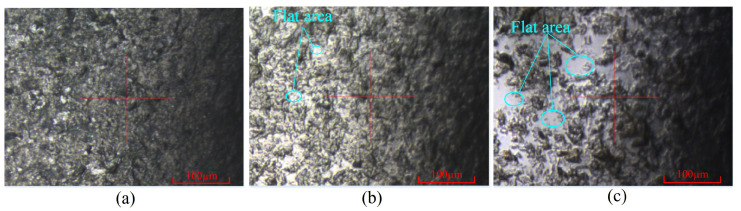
Two-dimensional surface morphology formed under different ultrasonic vibrations. (a) Original surface. (b) Ultrasonic longitudinal vibration. (c) Ultrasonic longitudinal–torsional vibration.
